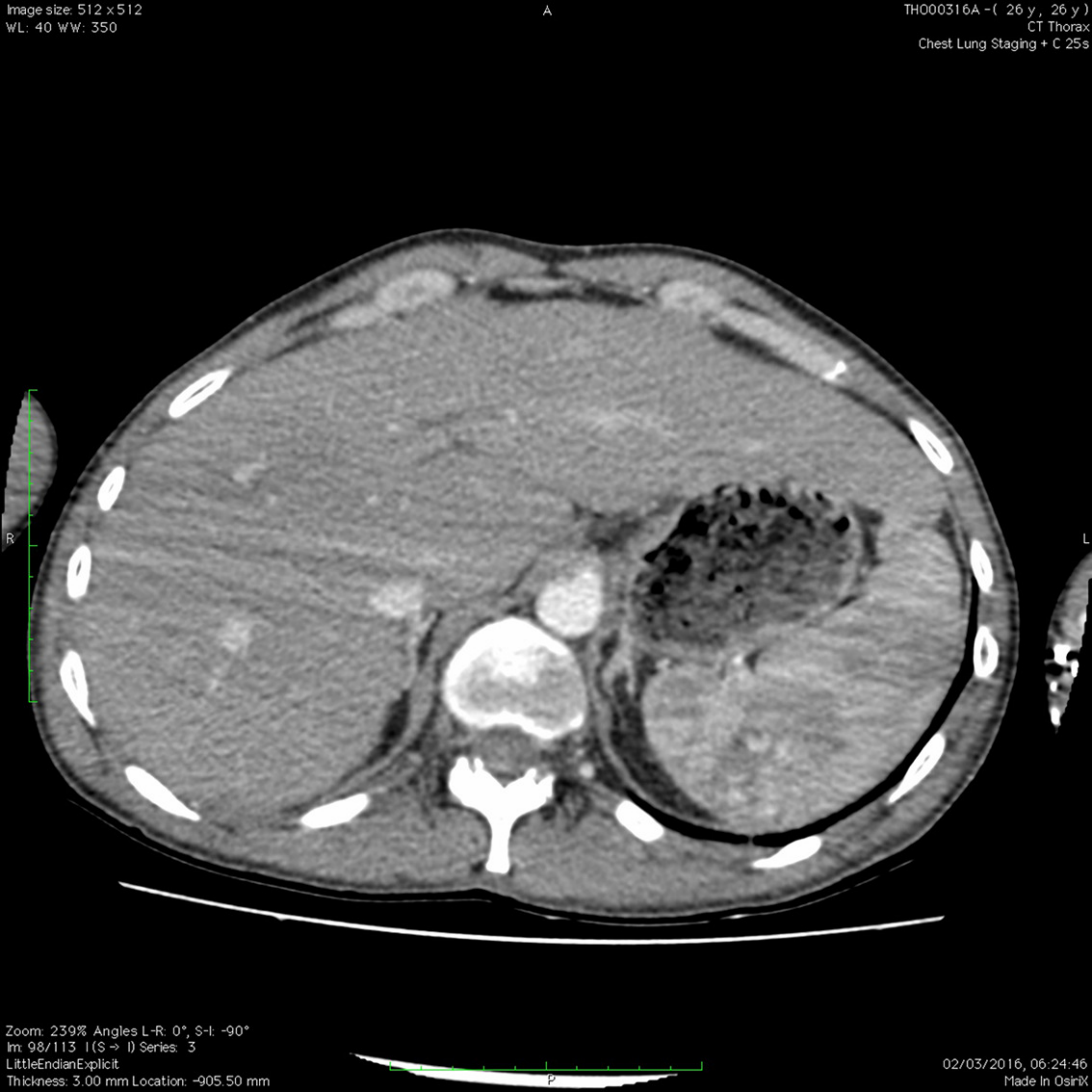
Axial view of the initial CT scan of thorax, acquired at 30s, showing subtle perfusion abnormalities within the spleen.
